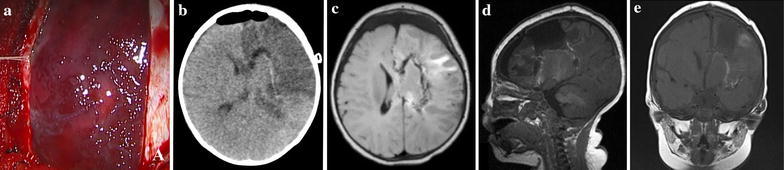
a Intraoperative image demonstrating leptomeningeal angioma; b Postoperative CT scan showing brain edema; c, d, e Postoperative MR images (axial, sagittal and cornonal view) confirming complete disconnection
